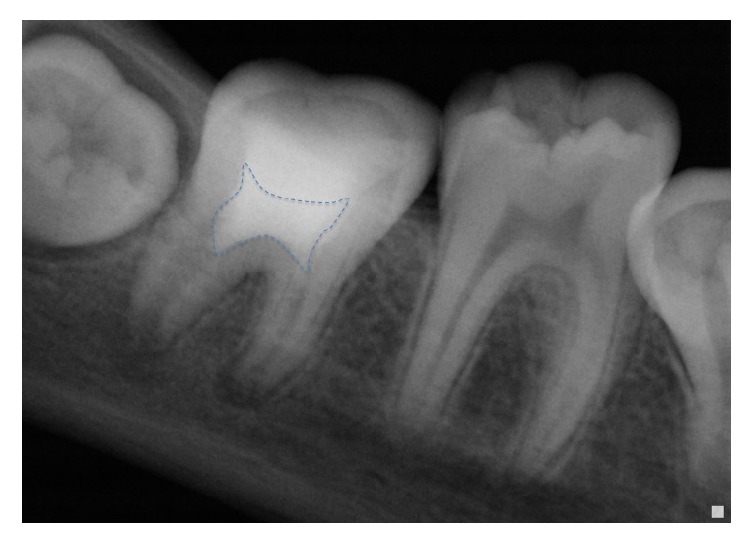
Control radiograph after capping with MTA.
